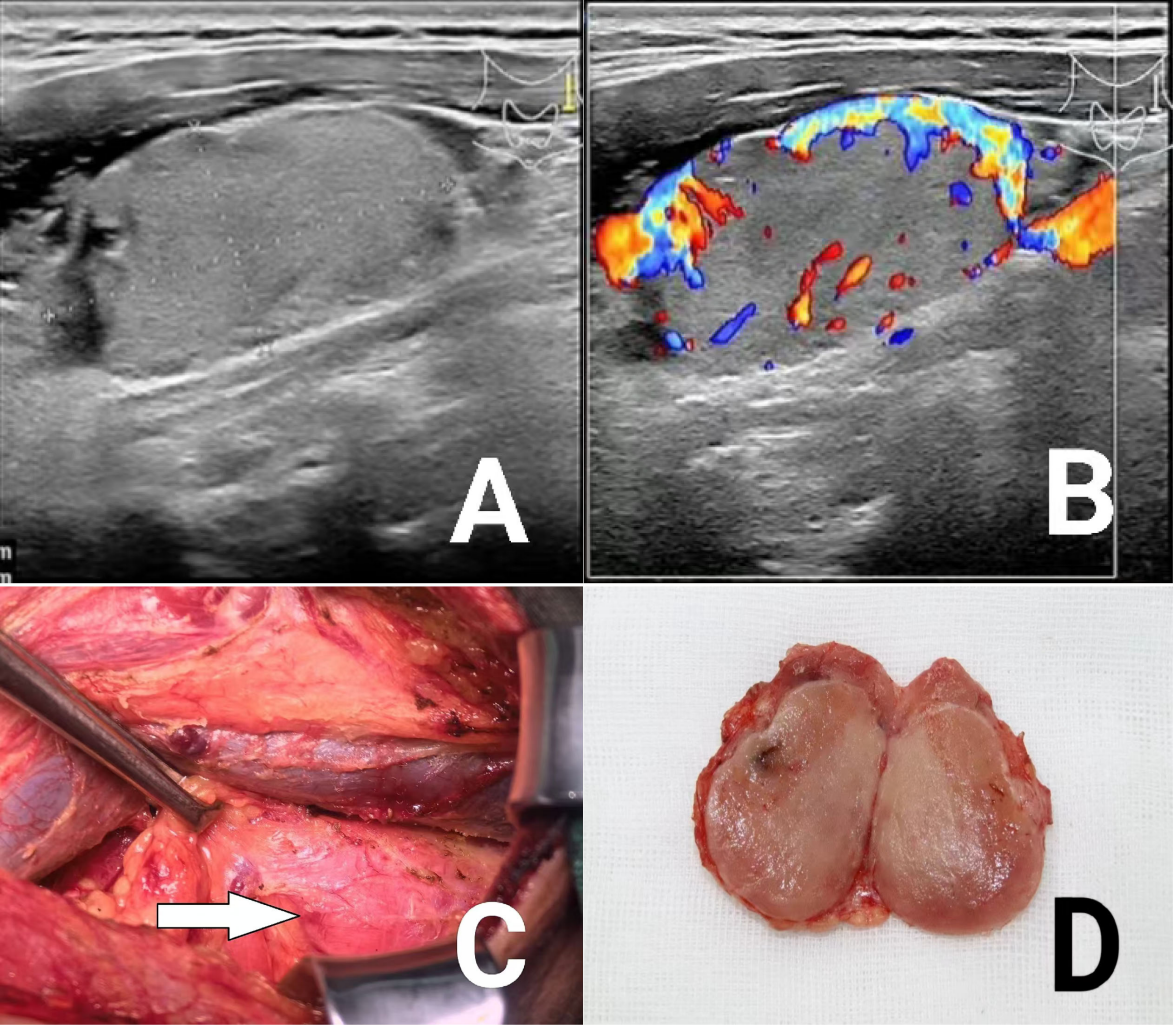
(A) Ultrasound image of lateral neck mass; (B) Ultrasound blood flow signal image of lateral neck mass; (C, D) Intraoperative and gross view of cervical masses.
